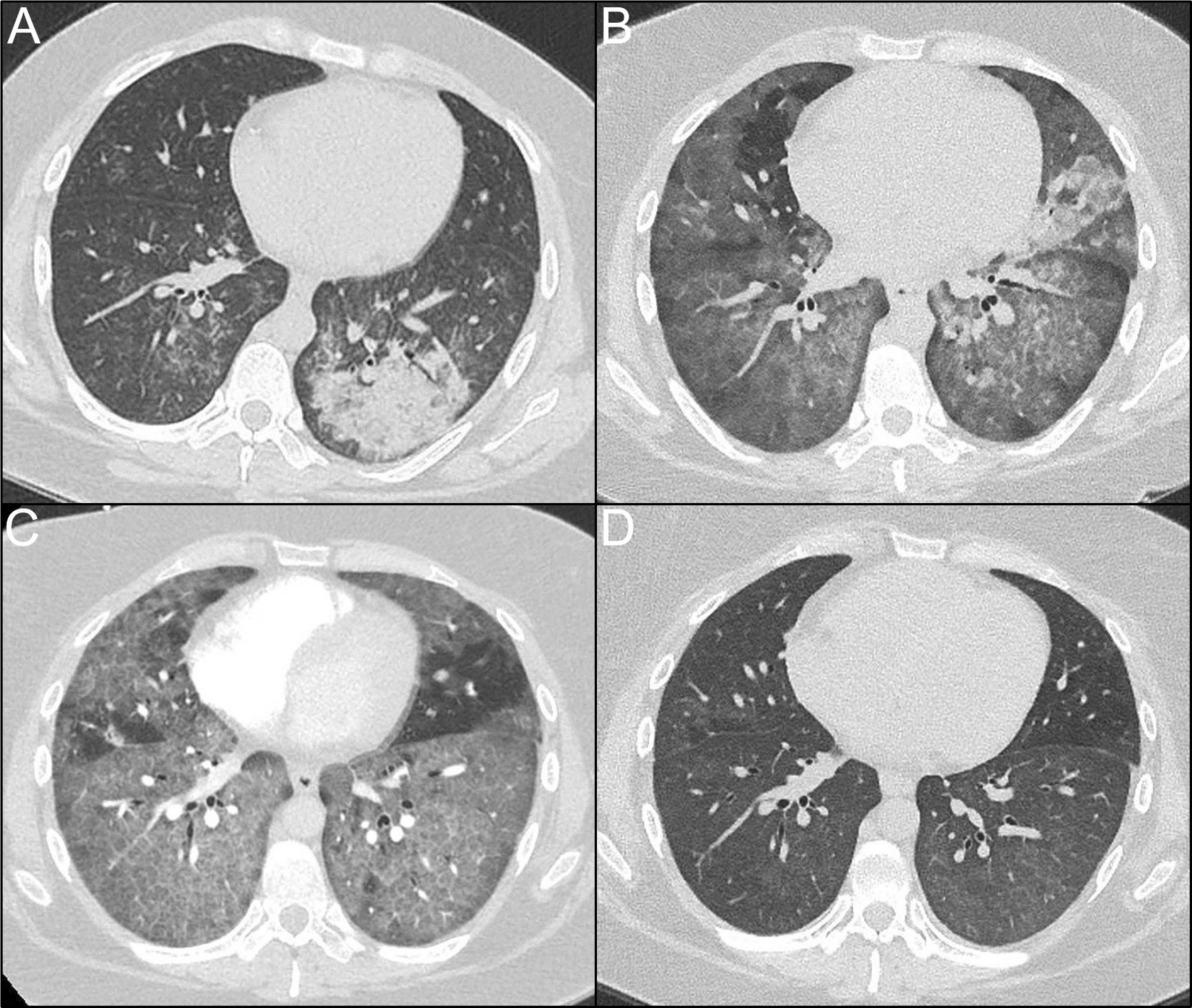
CT chest axial slices from initial presentation through to definitive management with whole lung lavage. (A) initial presentation with cryptococcal pneumonia with predominant left lower lobe consolidative change. (B) two weeks after initial presentation with progressive bilateral ground glass changes and early interlobular septal thickening which correlated with high CMV titre in BAL fluid. (C) Six weeks after initial presentation with progressive, confluent bilateral ground glass change and interlobular septal thickening in keeping with crazy paving pattern. (D) Four days post‐whole lung lavage with marked radiographic improvement.
